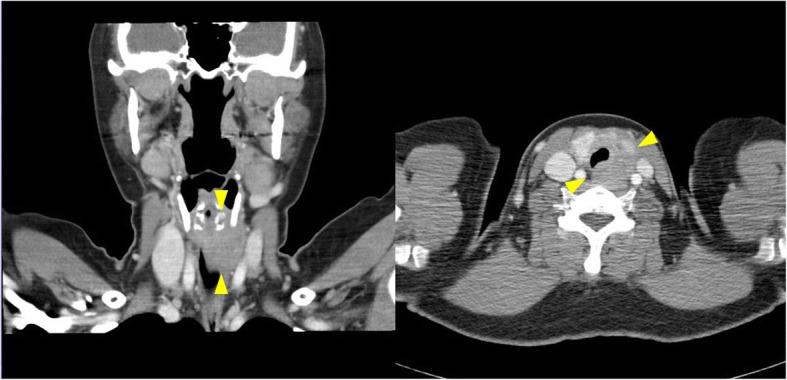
CT scan revealed a 4 cm hypodense mass with ill-defined margin at posterior-lower part of left thyroid gland (arrow heads), accompanied by multiple enlarged neck lymph nodes
